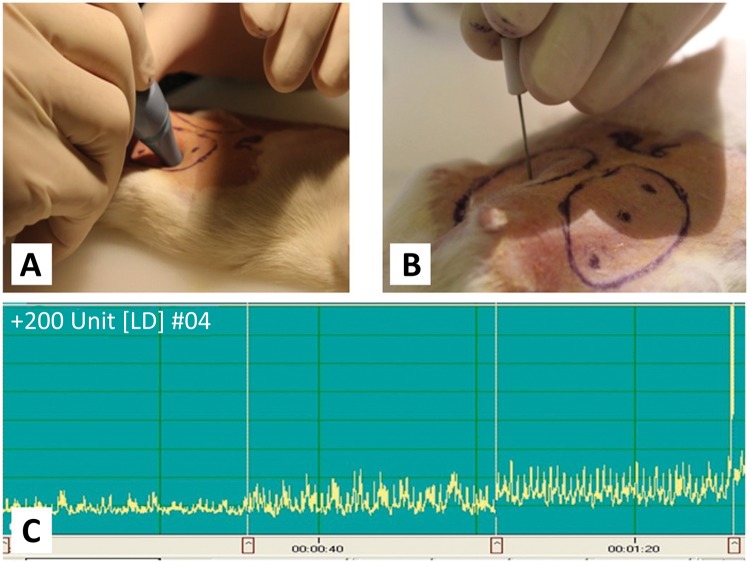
Using infrared thermometer (A) and laser Doppler pencil probe (B) taken on the defined sites of flaps. C: representative laser Doppler recording of the measurement sites (from left to right: distal, central, proximal).
